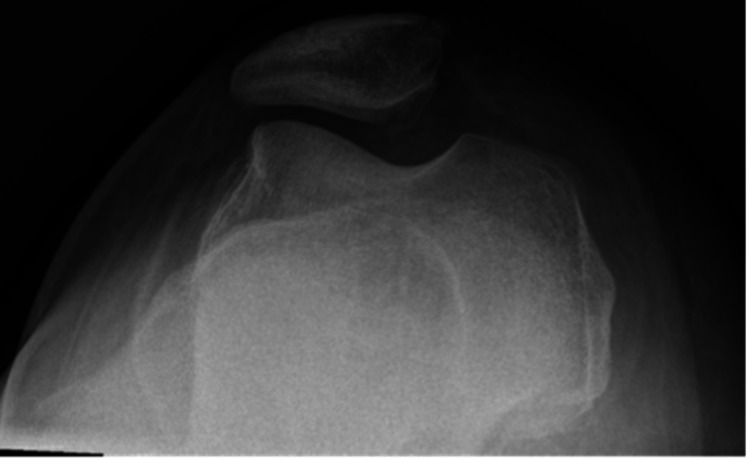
Comparison sunrise radiograph of the right knee showing laterally subluxated patella without congenital anatomic abnormality that may predispose the injured side to locked dislocation
